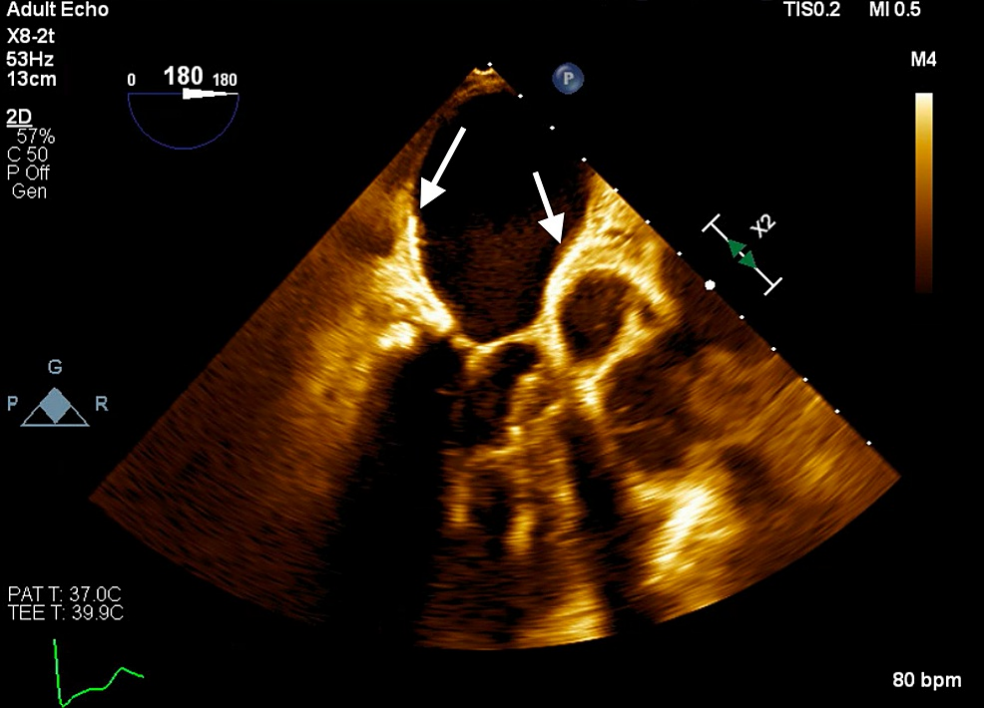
Transesophageal echocardiogram long axis view showing layering of highly echogenic material along the posterior left atrial wall.
